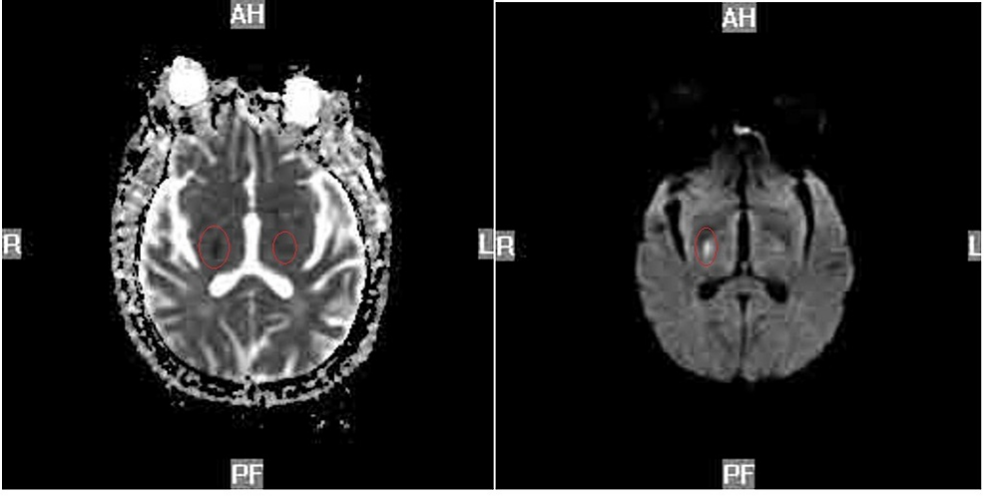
Diffusion-weighted MRI head (left). Axial MRI of the head (right) Note: Poor quality due to patient's hemiballismus
